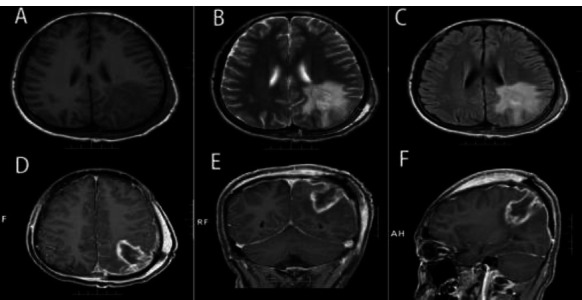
(A-F) The patient underwent an MRI examination one week after the surgery, and no tumor residue was found.
